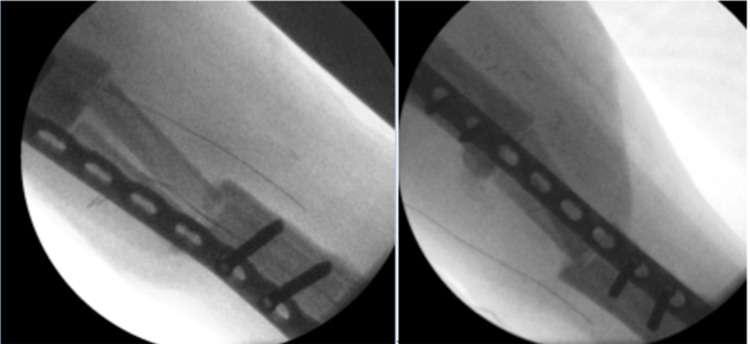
Intraoperative x-ray images of the fibula double barrel placement (April 2019)
